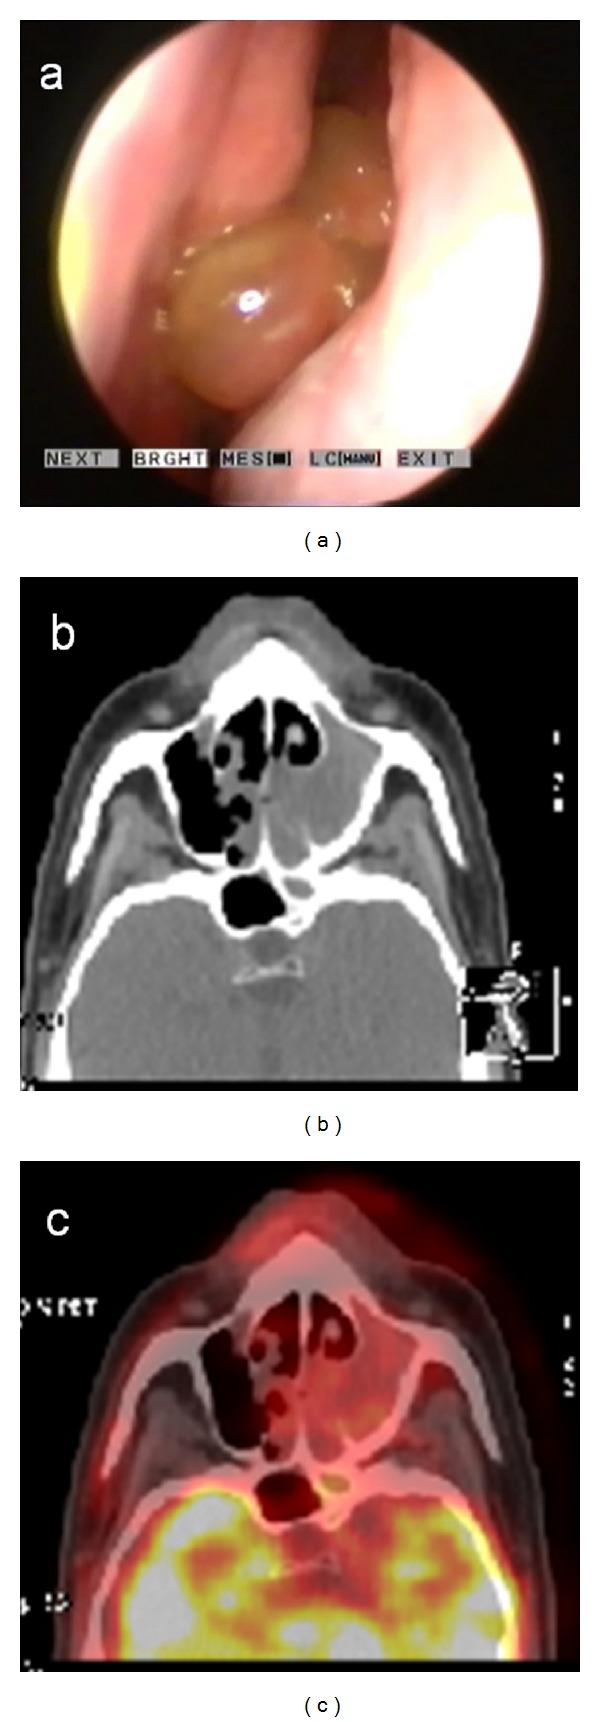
Case  7: (a) endoscopic view, (b) CT, and (c) ^18^FDG-PET/CT findings (SUV_max⁡_ 2.9).
